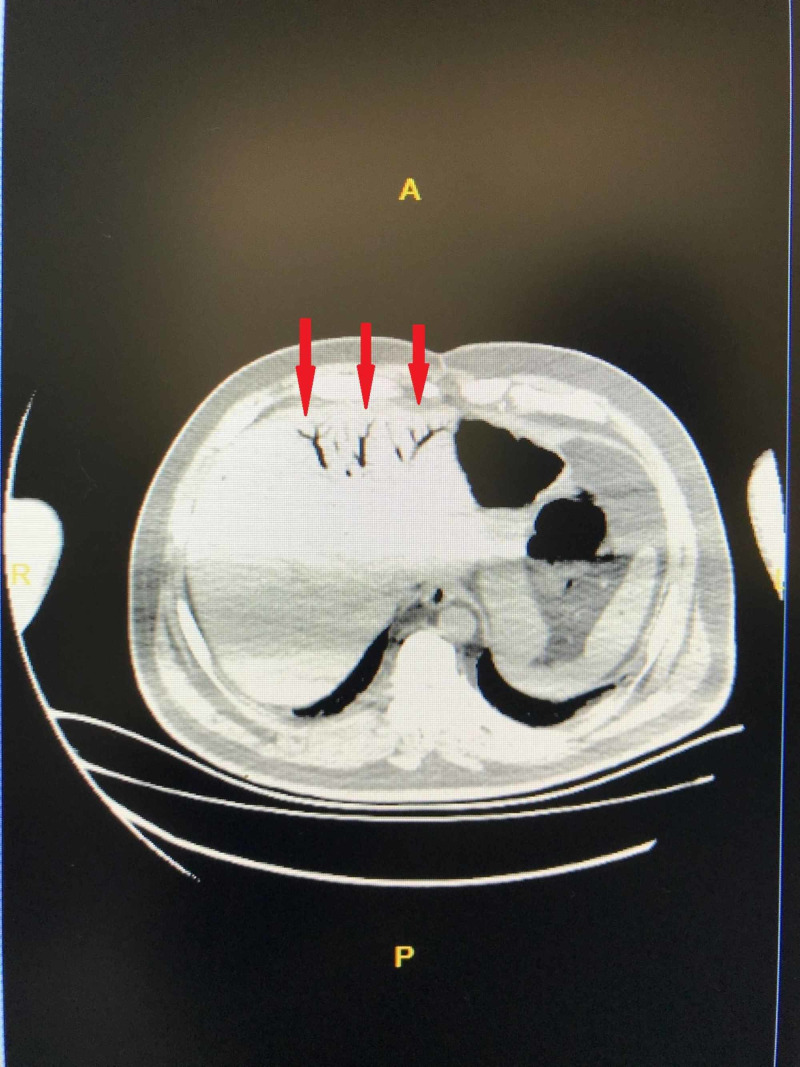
CT scan, axial plane Red arrows are showing the hepatic portal venous gas in the center and left  lobe of liver
